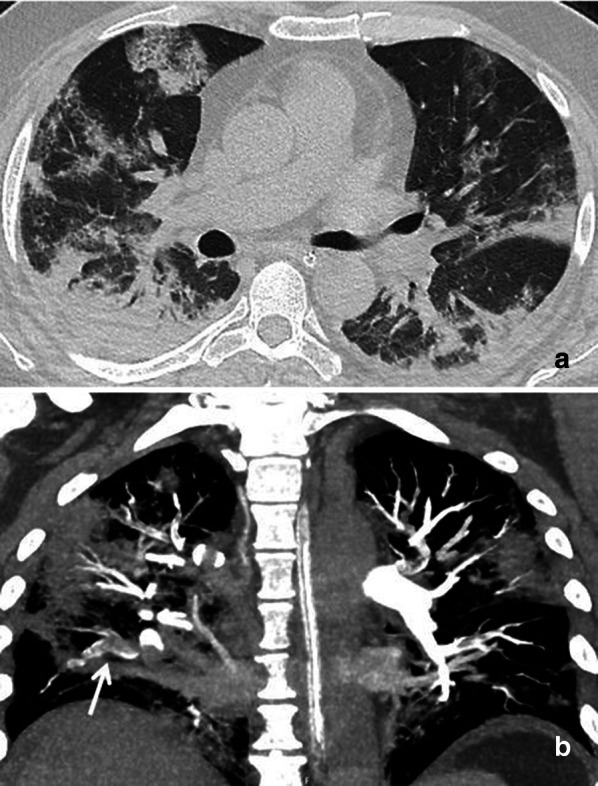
A 50-year-old man with a positive RT-PCR. a Axial CT image shows moderate COVID-19 pneumonia manifested by peripherally located GGO and consolidations. b Coronal pulmonary CT angiogram shows multiple filling defects in segmental and subsegmental pulmonary artery branches, clearly seen in the right inferior pulmonary branches (white arrow)
